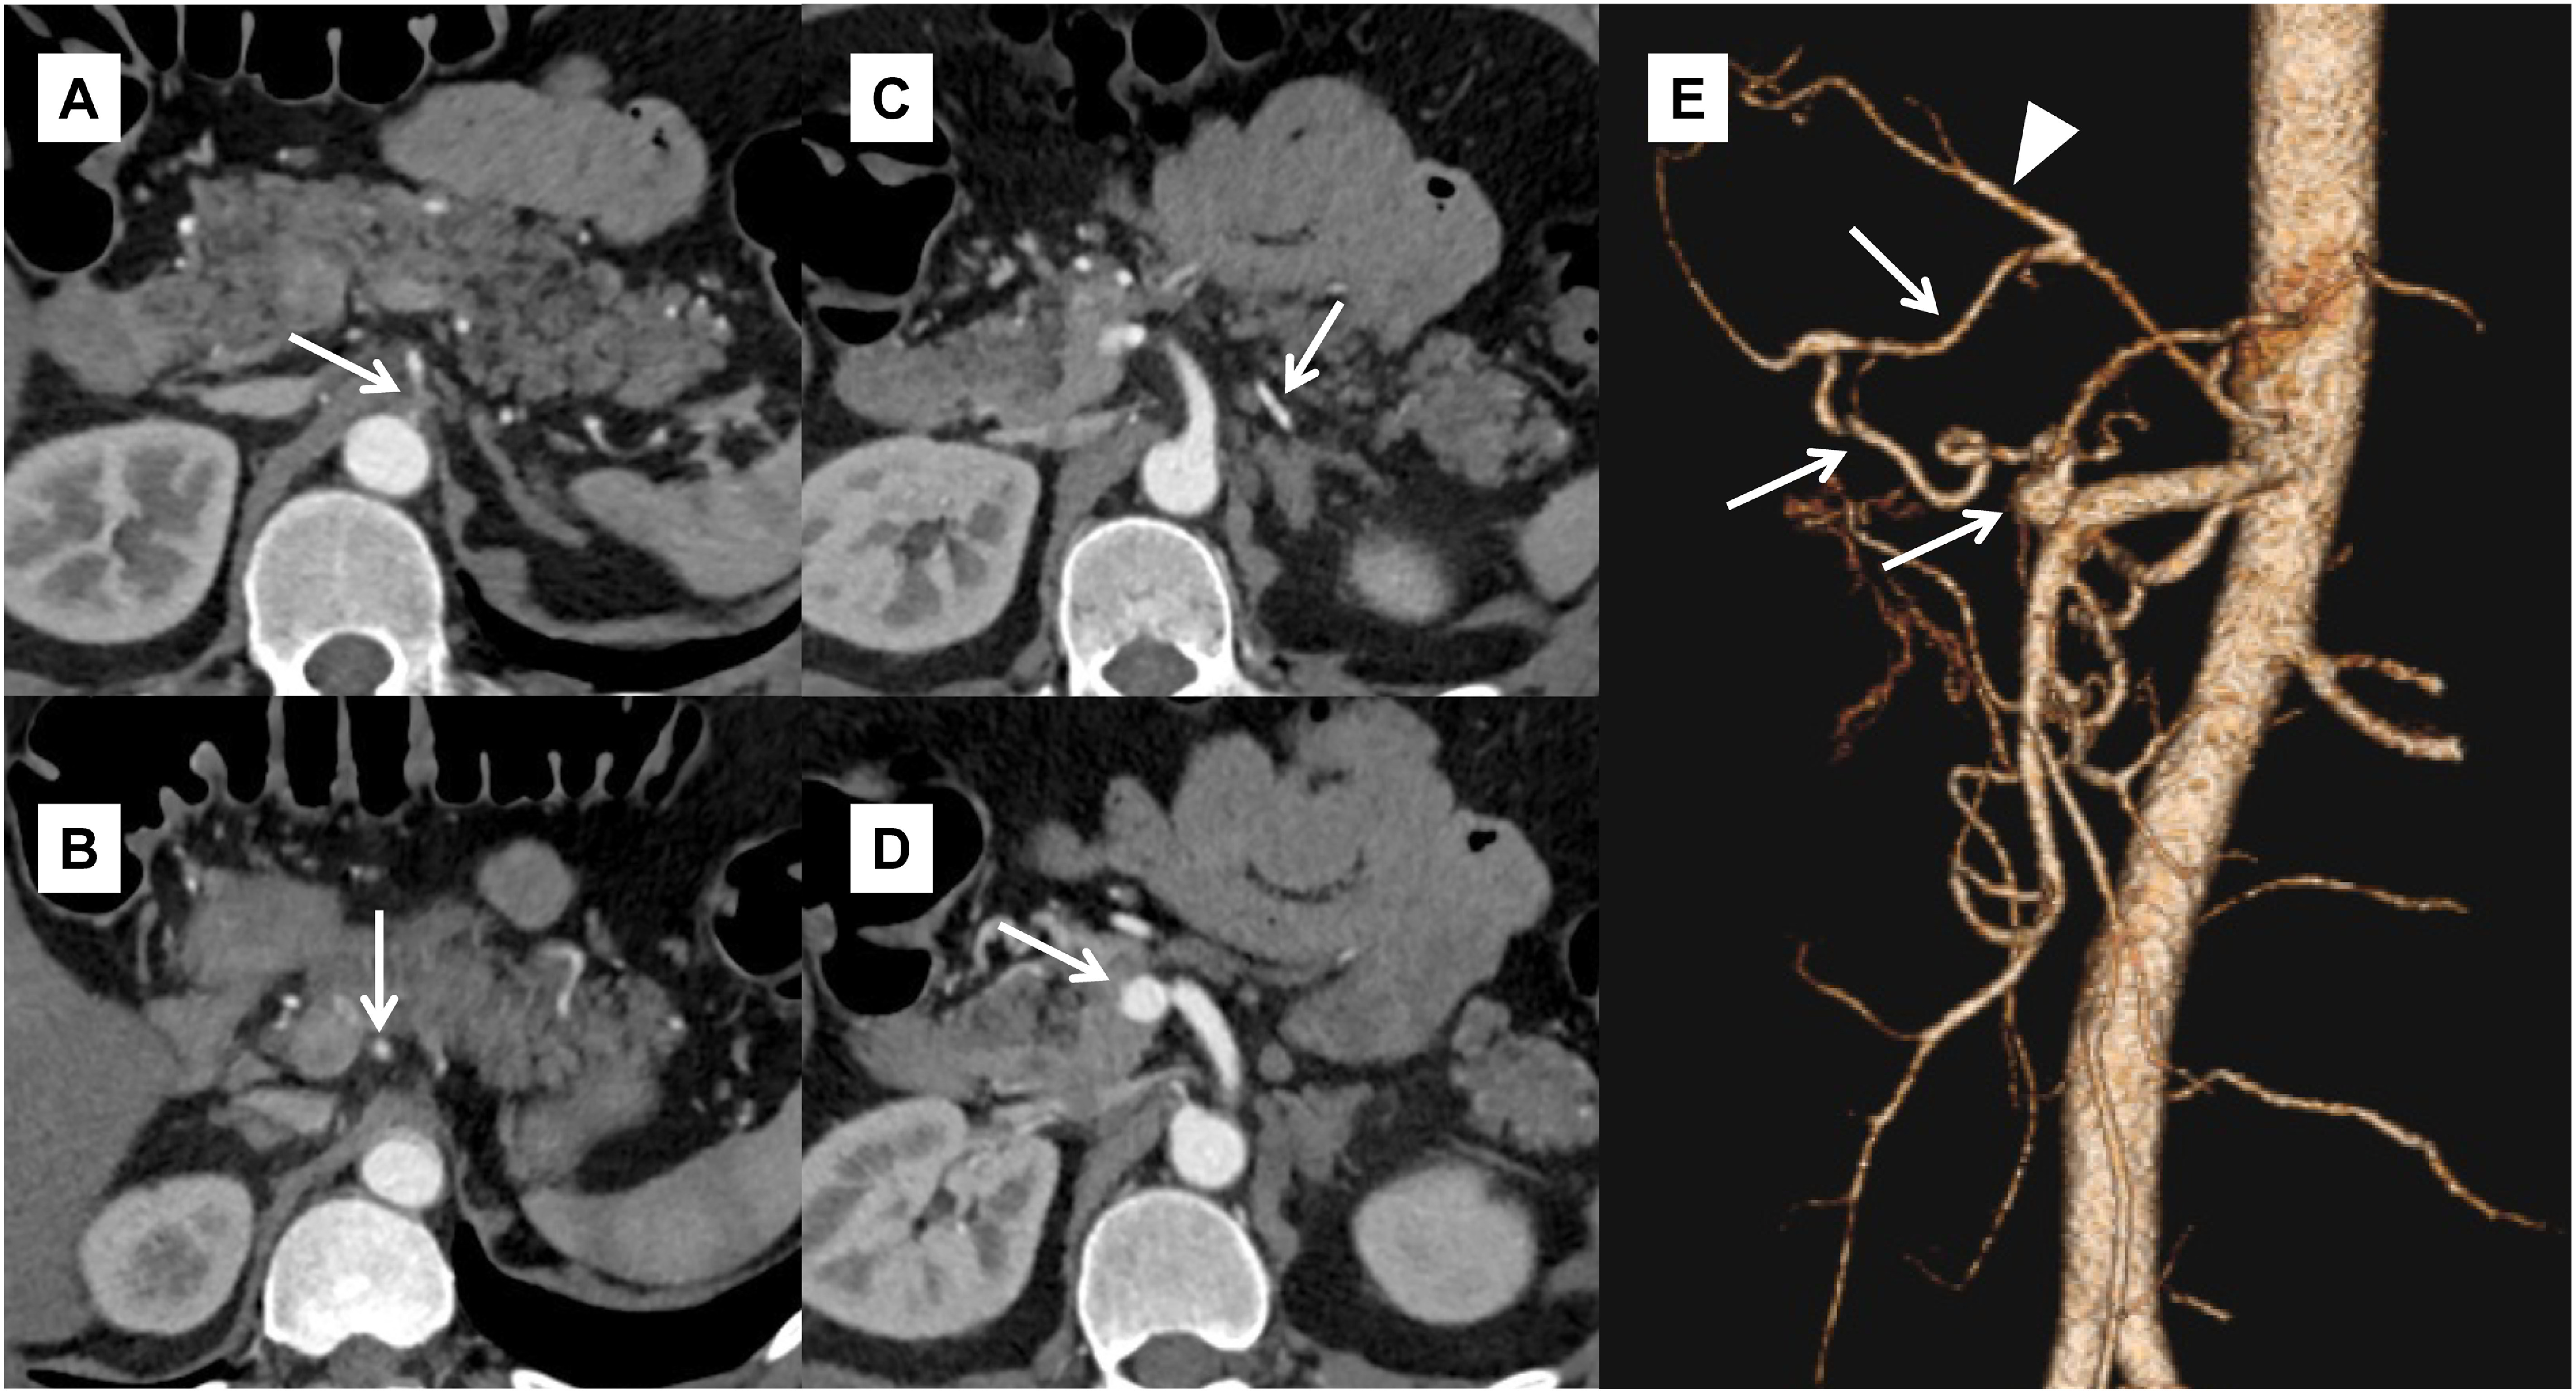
Fig. 1 Computed tomography (CT) reveals hypoplasia of the celiac axis (A, arrow), common hepatic artery (B, arrow), and splenic artery (C, arrow). The inferior pancreaticoduodenal artery (IPDA) aneurysm, 11 mm in size, branched from a dilated superior mesenteric artery, is close to the origin of the IPDA (D, arrow). Three-dimensional CT indicates the IPDA aneurysm with the development of pancreatic arcades (E, arrows), and the proper hepatic artery (E, arrowhead).
